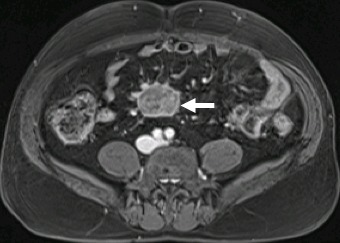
Axial T2 contrasted MRI, demonstrating delayed hyperenhancement and "tumor pseudocapsule"
